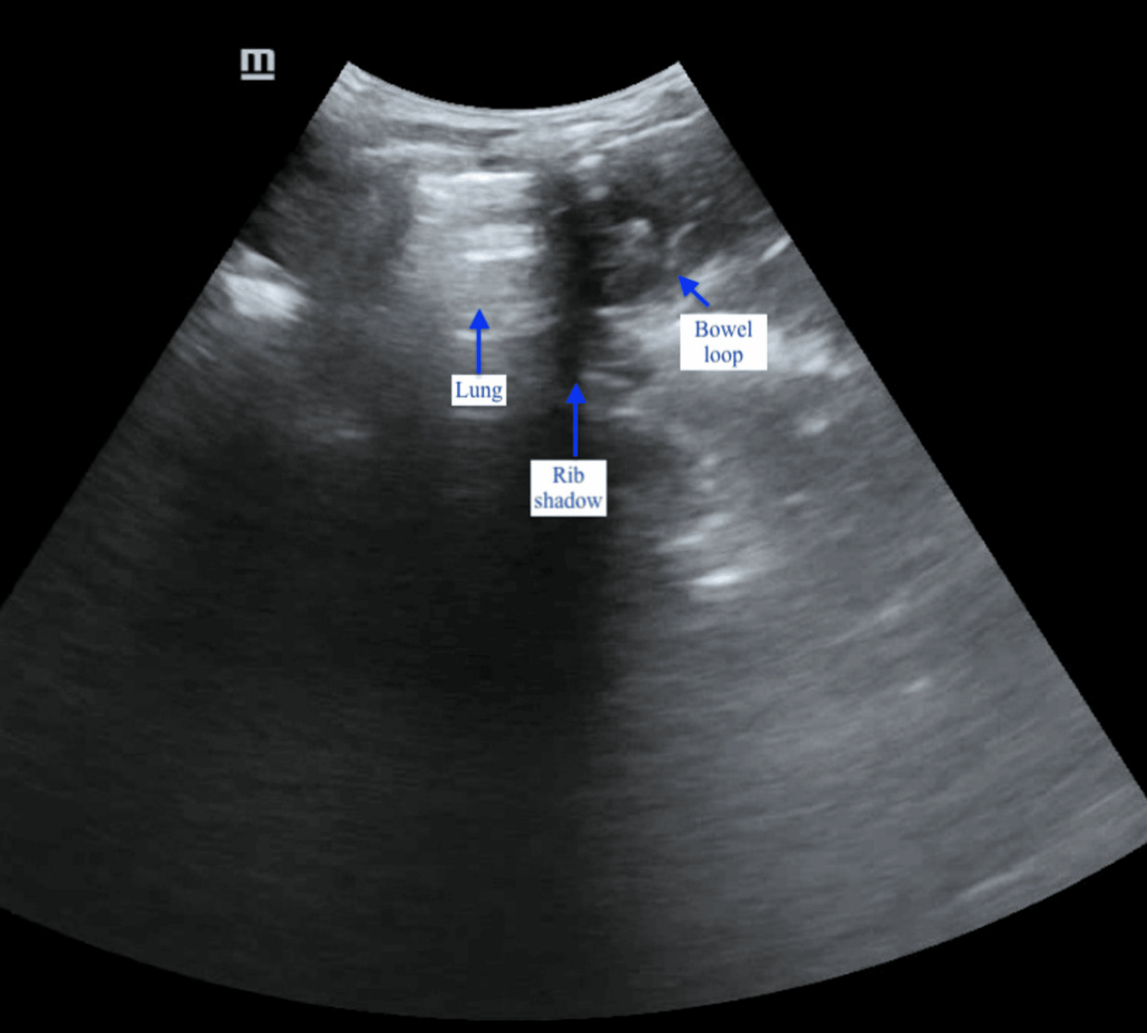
Ultrasound image of the left lung parenchyma (showing A-lines) on the left side of the rib shadow, with a bowel loop visible on the right side The probe marker was oriented toward the patient’s head.
